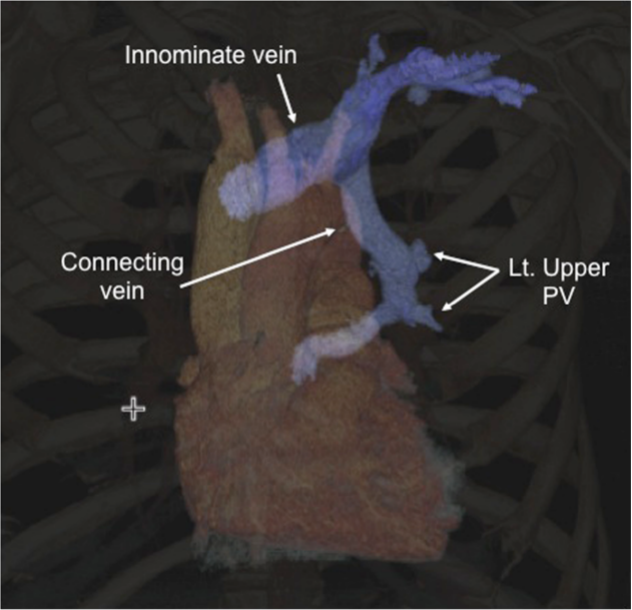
Preoperative computed tomography image. Three-dimensional CT showed left upper pulmonary vein connecting simultaneously to the innominate vein and the left atrium. PV, pulmonary vein.
